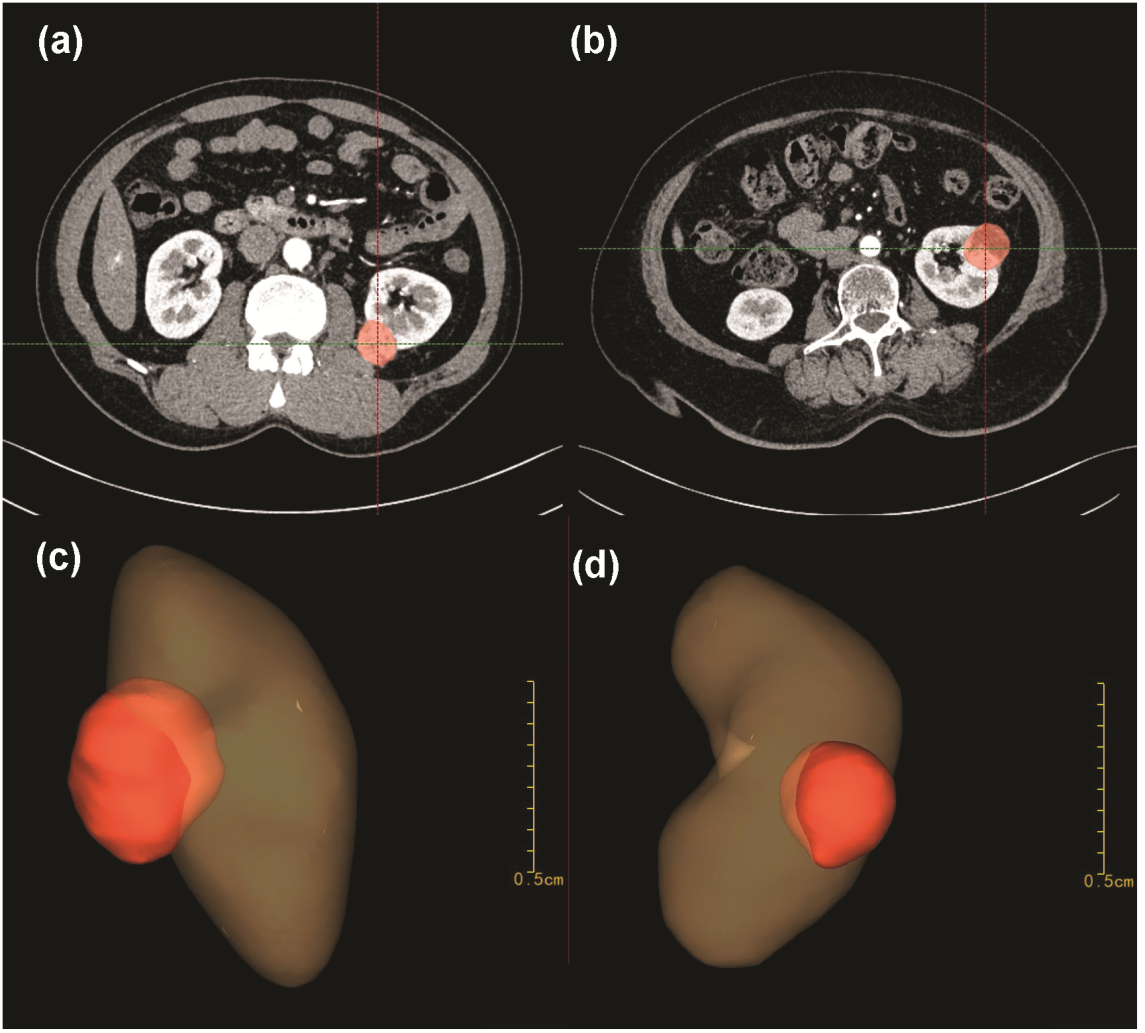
A longer 2D diameter was not equal to a larger 3D volume in our cases. Patient A measured a shorter diameter (A) in 2D CT images than patient B (B). After 3D reconstruction, patient A (C) measured a greater volume than patient B (D).
